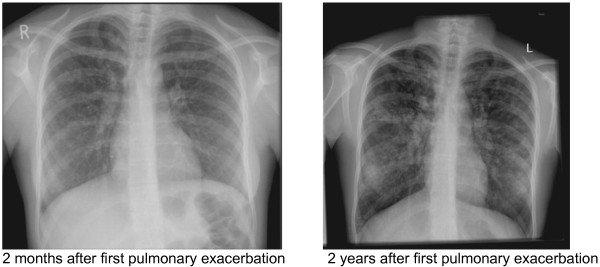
Chest X-ray of a patient (3) infected with *T. inkin *two months and two years after first pulmonary exacerbation due to *T. inkin*. The second X-ray demonstrates a strong increase in hyperinflation, increasing bronchiectasis and mucus plugging, increasingly thickened bronchial walls and numerous additional patchy lesions.
